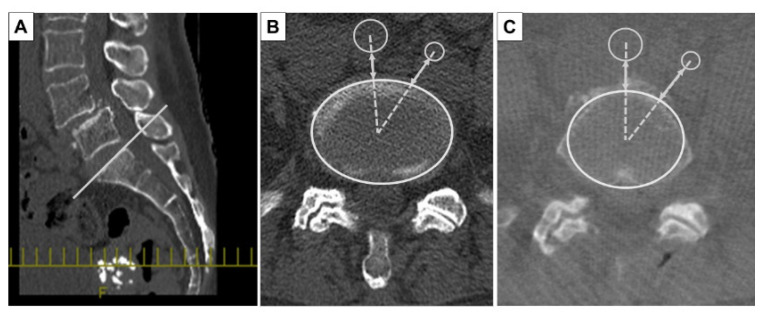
Measurement method of computed tomography (CT) images. Multi-planer reconstruction (MPR) Works software (GE Healthcare) was used to measure the shortest distance between the abdominal arteries and the vertebral body or intervertebral disc (SDA) at each level. When measuring the disc level, the MPR line was positioned in the sagittal plane at the cranial endplate of the caudal vertebral body (A), a line was drawn to connect the center point of the disc with the center point of the artery, and the shortest distance (two-way arrow) between the anterior margin of the disc and the artery wall margin was measured. Preoperative CT (B) and intraoperative CT (C) images were analyzed at each level precisely.
